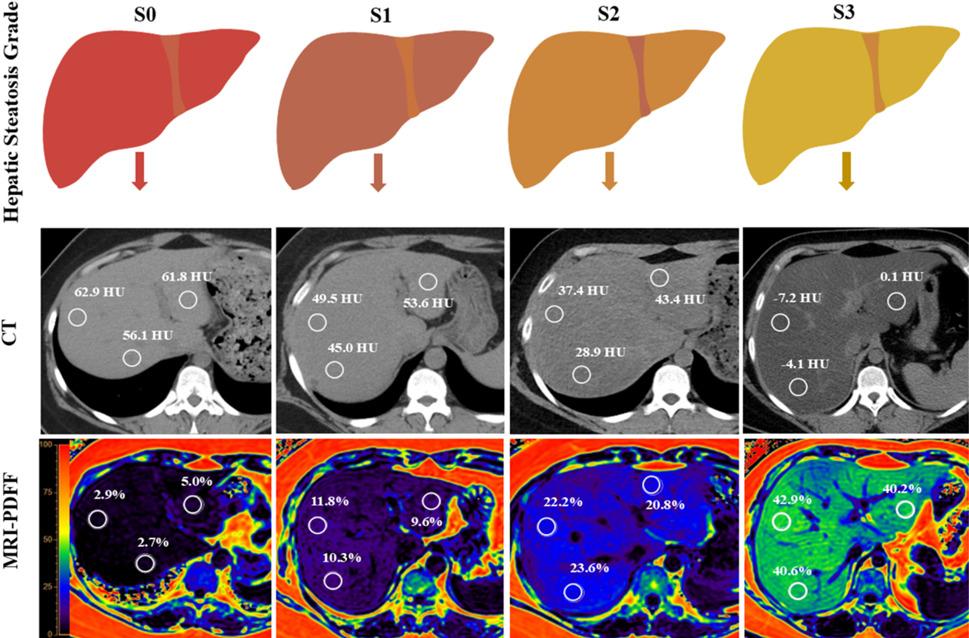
Non-contrast chest CT and liver MRI-PDFF images of pathologically confirmed different hepatic steatosis grades (S0 - 3). Three round ROIs were placed at the left lobe, anterior and posterior part of the liver right lobe, and the mean liver attenuation and PDFF value of the three ROIs were taken as the liver fat content.
